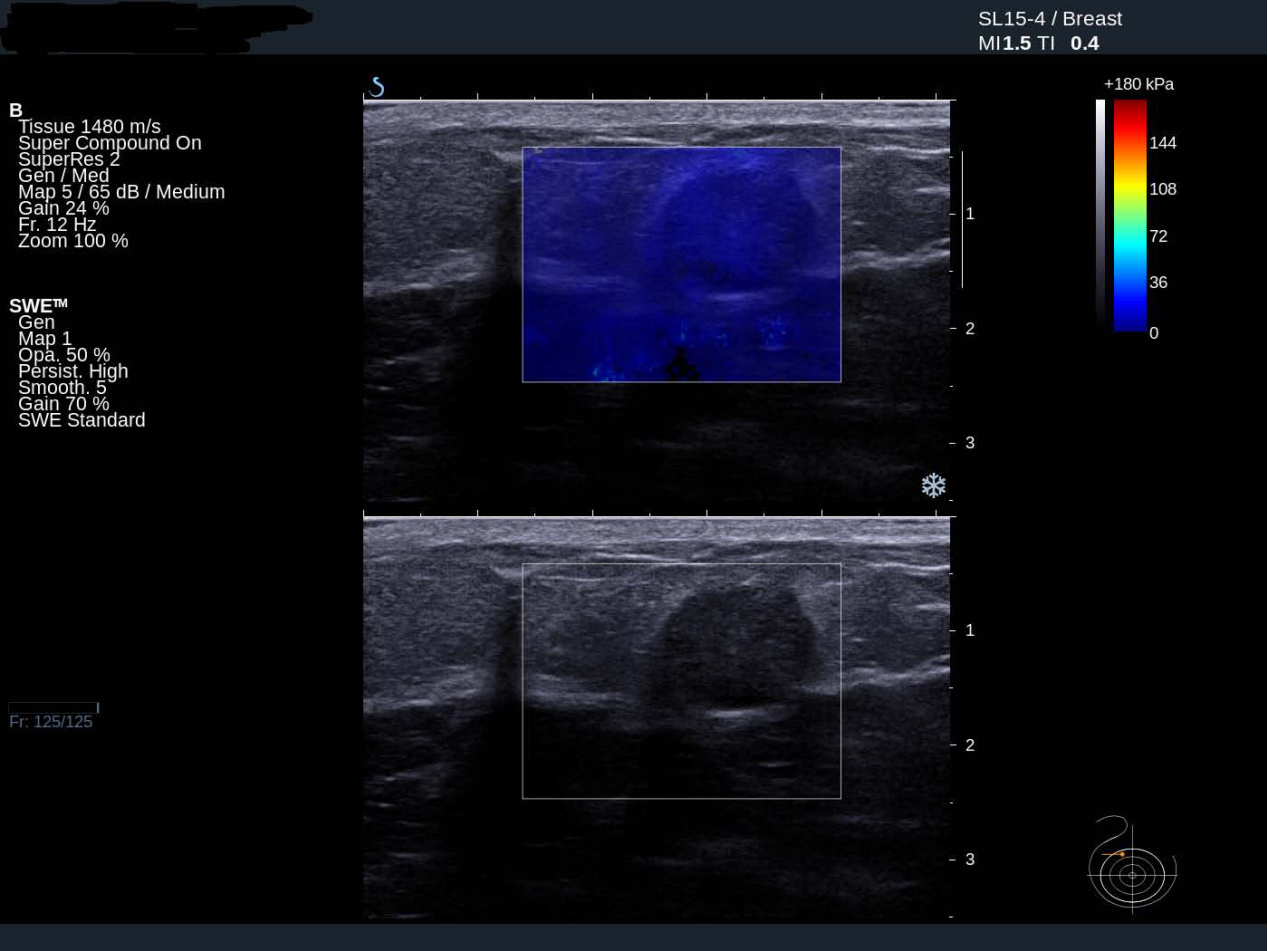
Ultrasound and elastography images of a fibroadenoma showing low stiffness. Mean 25 kPa.
